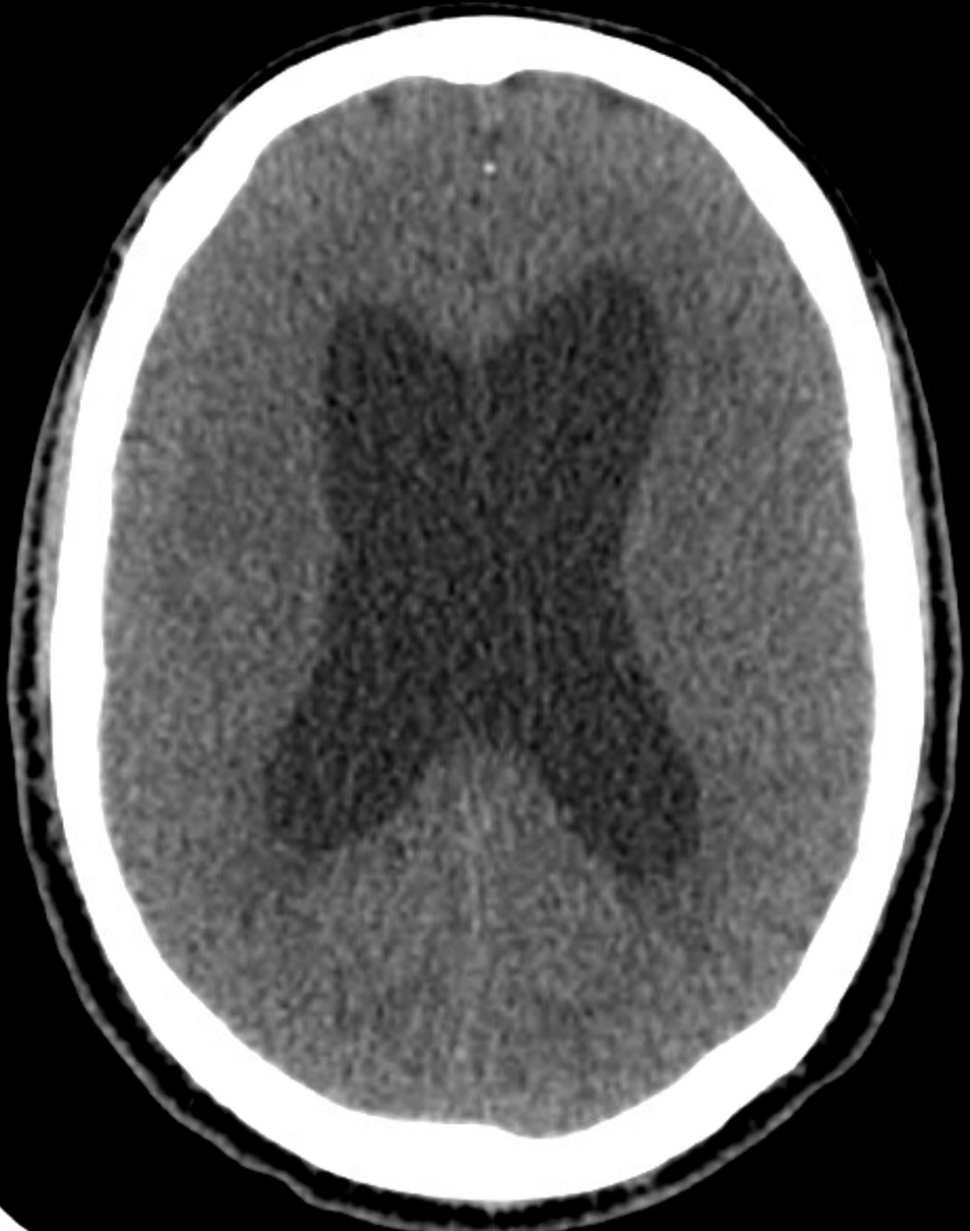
Brain CT showing ventriculomegaly CT: computed tomography
